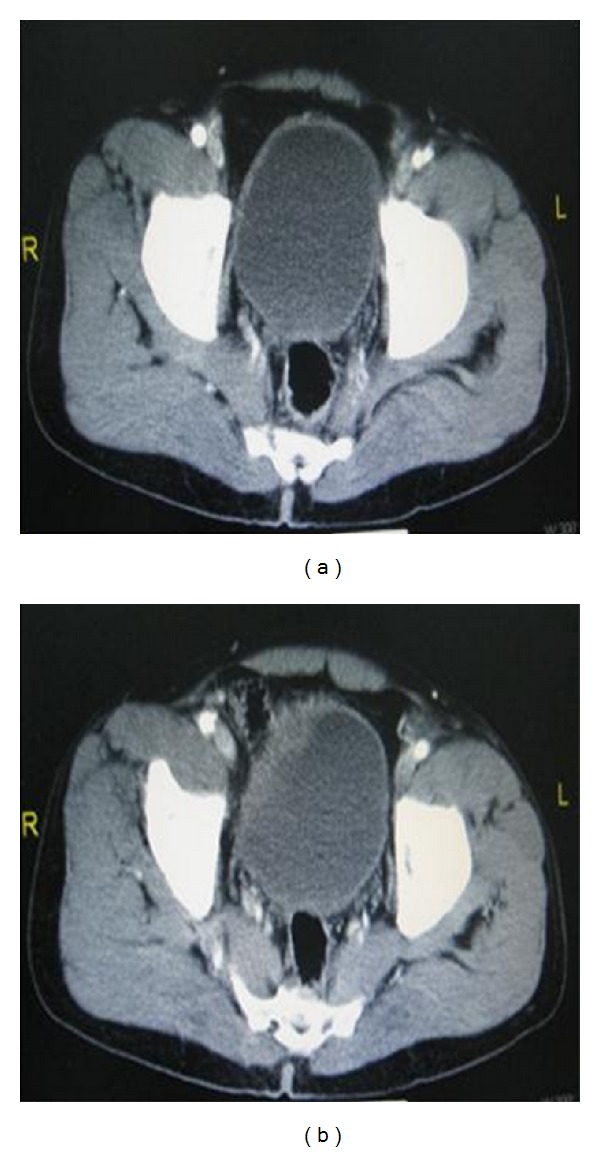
(a) CT image outlines the distended urinary bladder (UB). (b) CT image showing irregularity and thickening on the right side of UB wall.
